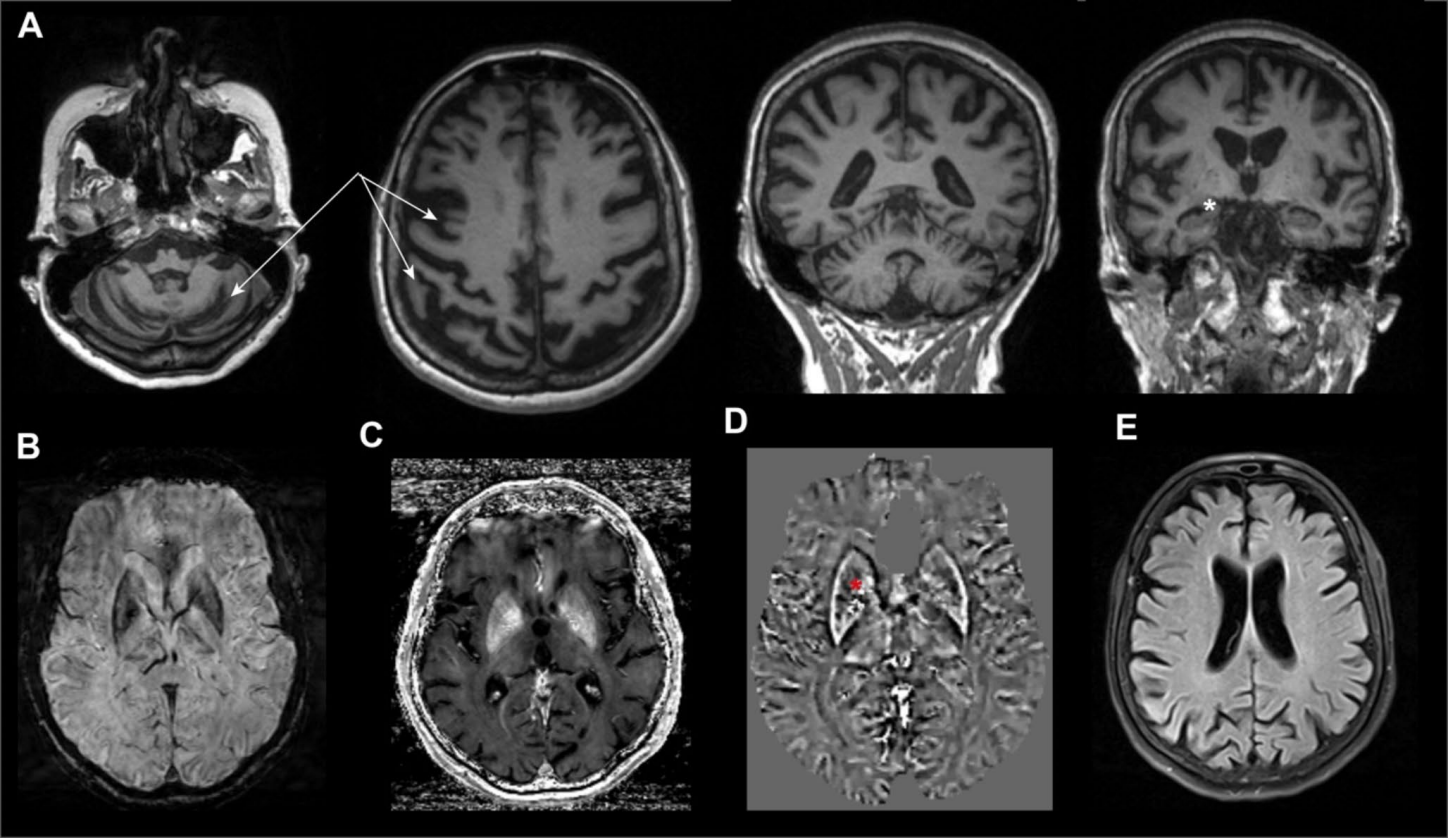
A T1w MPRAGE shows pronounced cerebellar and fronto-parietal atrophy (white arrows) with normal hippocampal volume (white asterisk). B Susceptibility weighted imaging (SWI) shows regular configuration of the basal ganglia without caudate atrophy. C R2* mapping and D quantitative susceptibility mapping revealed iron deposits in a rim-like fashion in the putamen and in the internal segment of the globus pallidus with minor diamagnetic signal (i.e., Ca^2+ −^signal; red asterisk). E FLAIR imaging shows no evidence of white matter pathology
